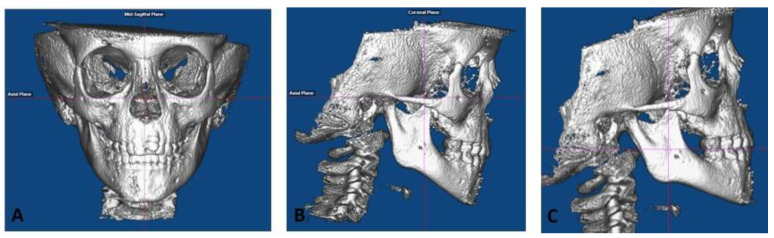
CBCT Orientation (A) Roll and Yaw, (B) Pitch (non-tooth-bearing, skeletal measurements), and (C) Pitch (tooth-bearing, dentoalveolar measurements).
